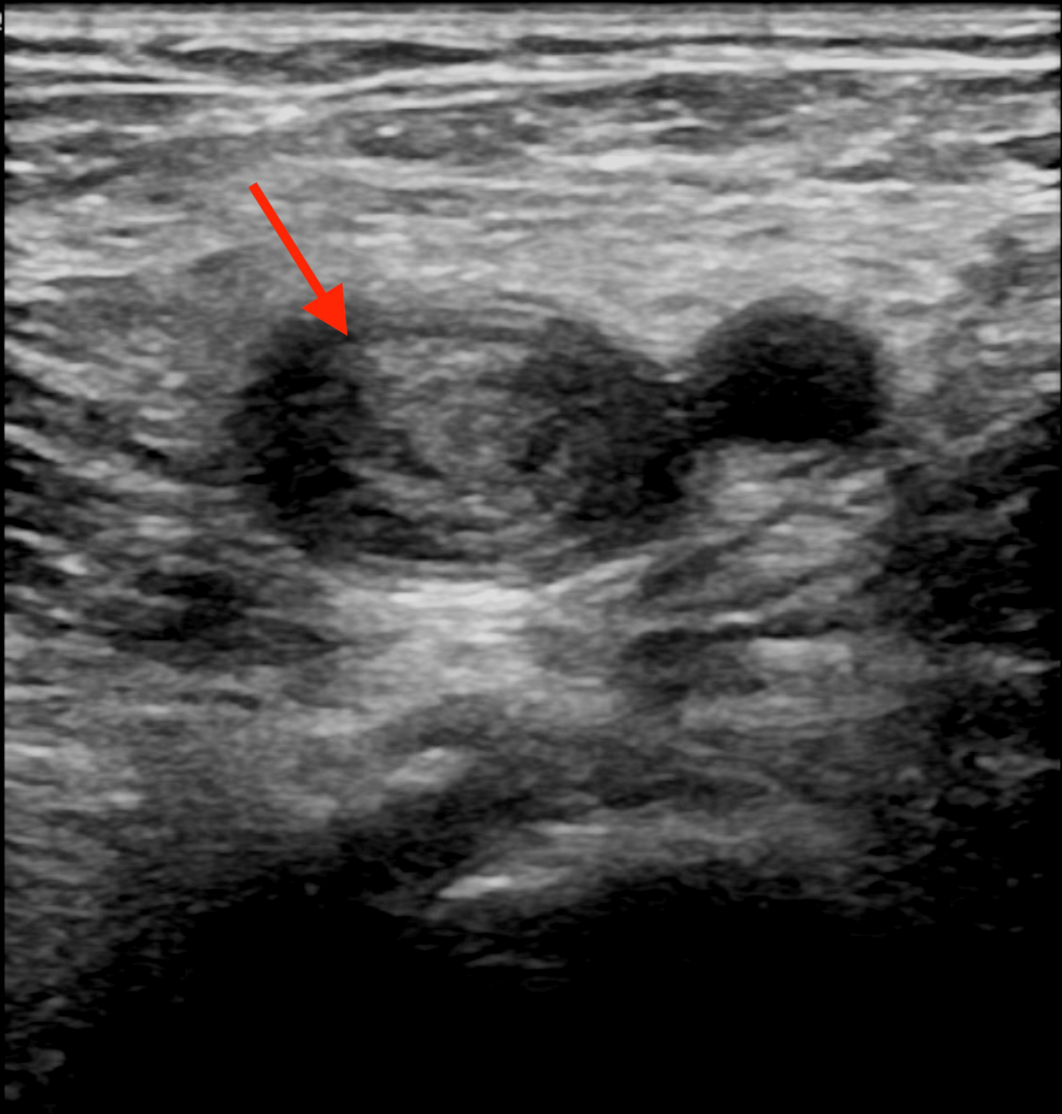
Compression ultrasound demonstrating a non-compressible, hyperechoic thrombus (red arrow) occupying the entire lumen of the left common femoral vein
